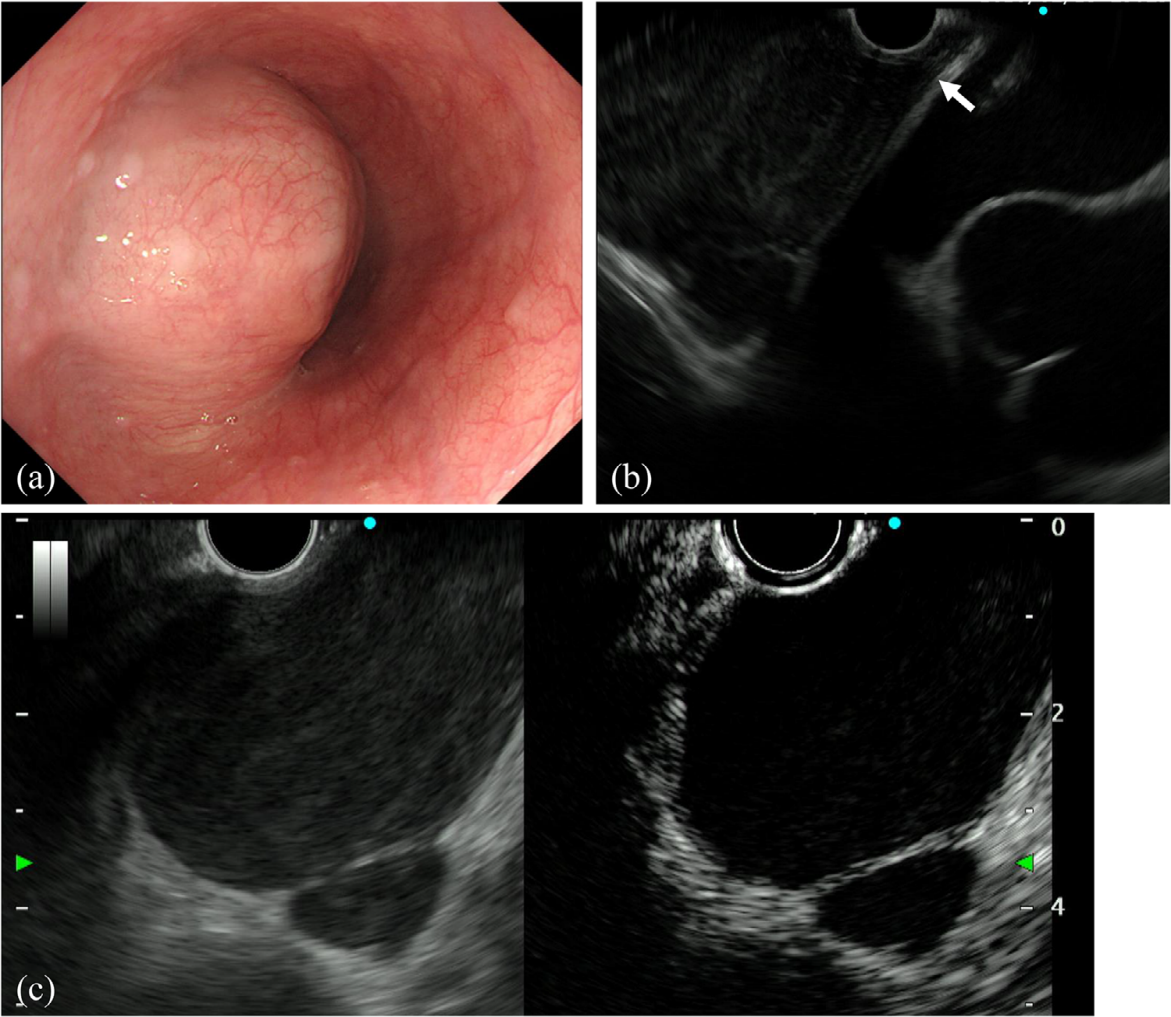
(a) A large mass with a gentle elevation and no epithelial changes causing narrowing of the lower esophageal lumen on esophagogastroduodenoscopy. (b) A hypoechoic lesion contiguous with the intrinsic muscular layer of the esophagus (arrow) is observed on endoscopic ultrasonography (7.5 MHz). (c) Multifocal hypoechoic areas are delineated in the anechoic area with a uniform wall structure on contrast‐enhanced harmonic ultrasonography (7.5 MHz).
